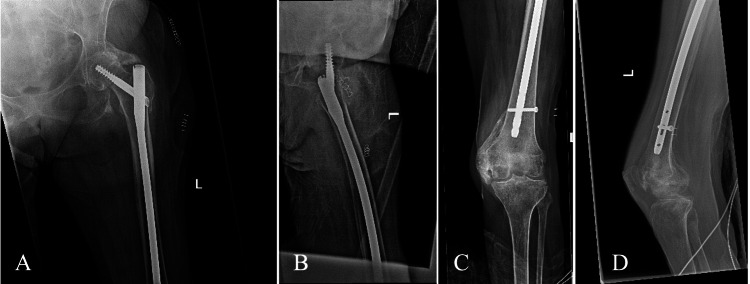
Immediate postoperative radiographs of the left femur demonstrating fixation of the left IT femur fracture with an unreamed intramedullary long nail A) Anteroposterior view of the left proximal femur. B) Lateral view of the left proximal femur. C) Anteroposterior view of the left distal femur. D) Lateral view of the left distal femur. IT: Intertrochanteric
